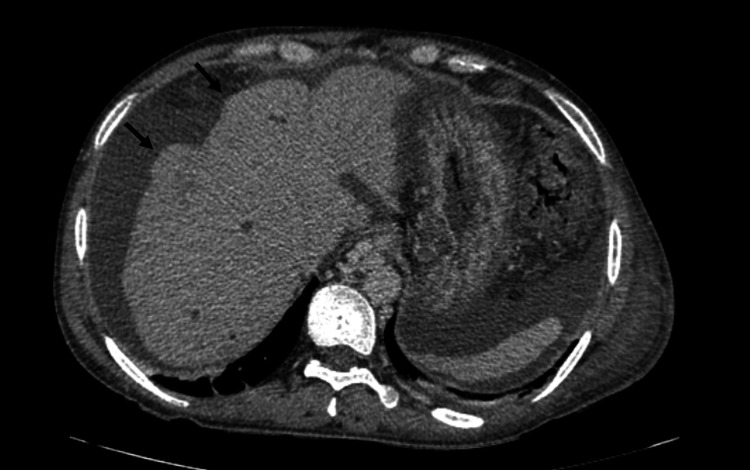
Three-phasic CT scan in portovenous phase of a 57-year-old male, which is a known case of PSC, referred for liver transplantation. The lobulated border is observable. PSC: primary sclerosing cholangitis
